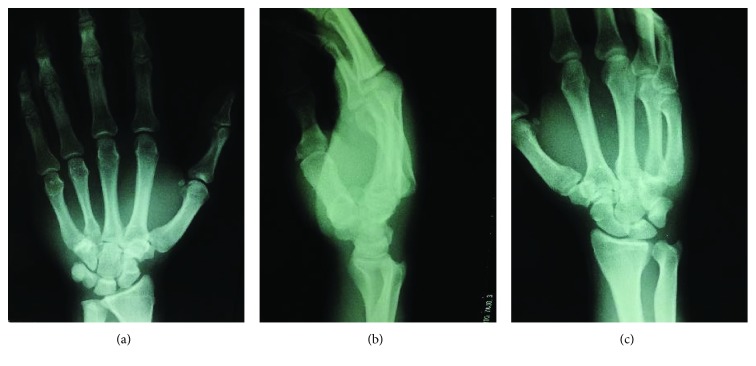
Anteroposterior (a), lateral (b), and internal oblique (c) radiographs of the right hand showed dorsal dislocation of the fourth and fifth CMC joints, without fracture.
